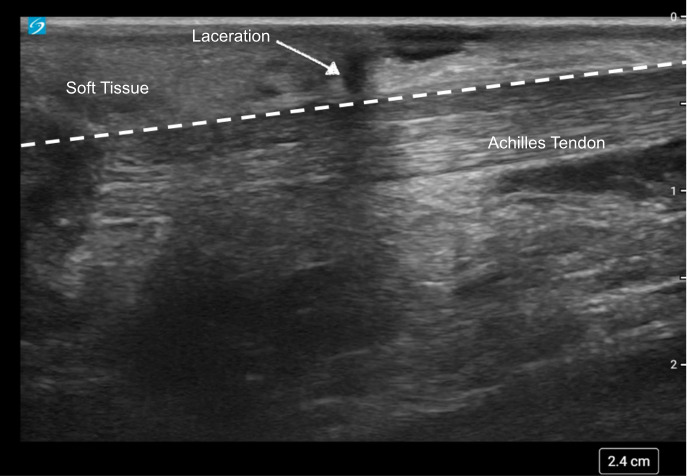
Point of care ultrasound (POCUS) image showing a sagittal view of the Achilles tendon (below the dotted line) with a laceration (arrow) above, but not including, the tendon.
